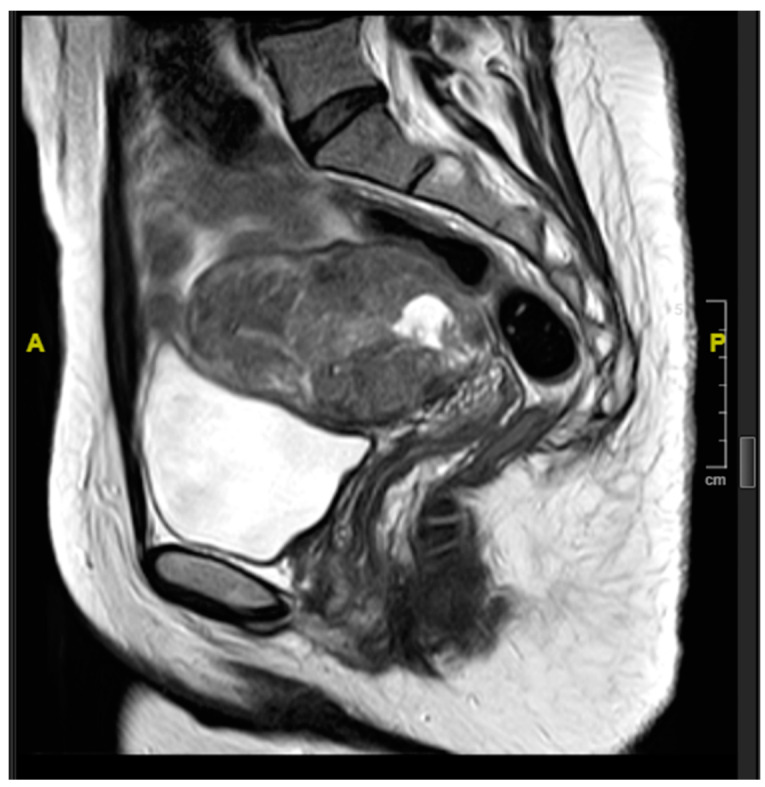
Sagittal T2-weighted MRI showing mass centred on the lower uterine segment with associated uterine distortion.
